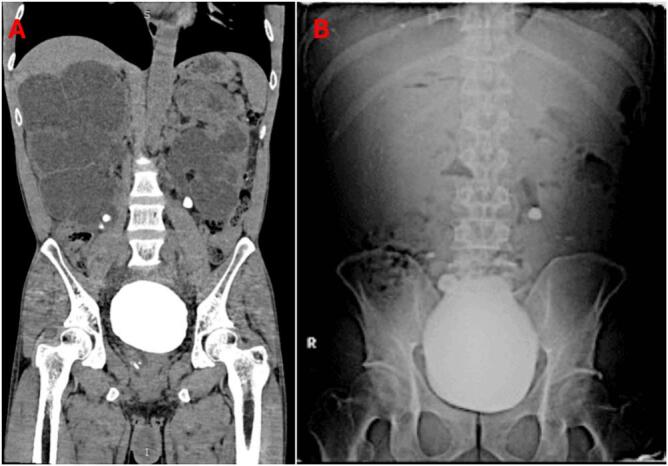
(A) CT sonography and (B) Abdominal plain radiograph.
